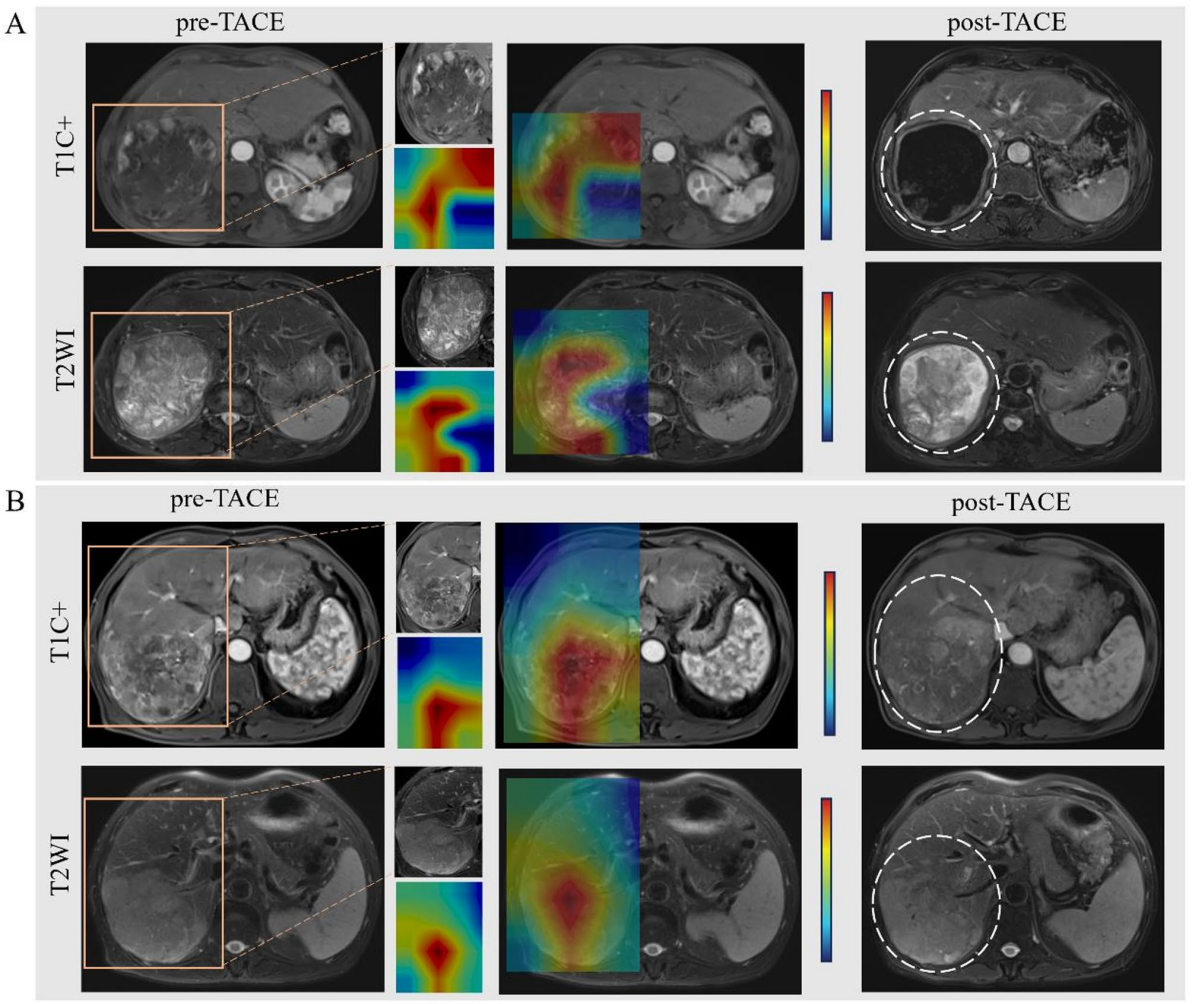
Representative cases of the attention map of (A) HCC with objective response to TACE, and(B) HCC with non-objective response to TACE. A A 72-year-old male HCC patient with a lesion diameter of 115 mm underwent MR scan 2 weeks before TACE. MR scan was re-examined 44 days after TACE. According to CE-MRI and T2WI images, the lesion diameter was similar to that before, but 95% necrosis occurred, so its TACE efficacy was evaluated as complete response (OR). B A 69-year-old male HCC patient, with a lesion diameter of 102 mm, underwent MR scan before (1 week) and after (31 days) TACE. The lesion diameter was stable and most of the lesions were viable. Hence, the corresponding early efficacy of TACE was evaluated as stable response (NOR)
